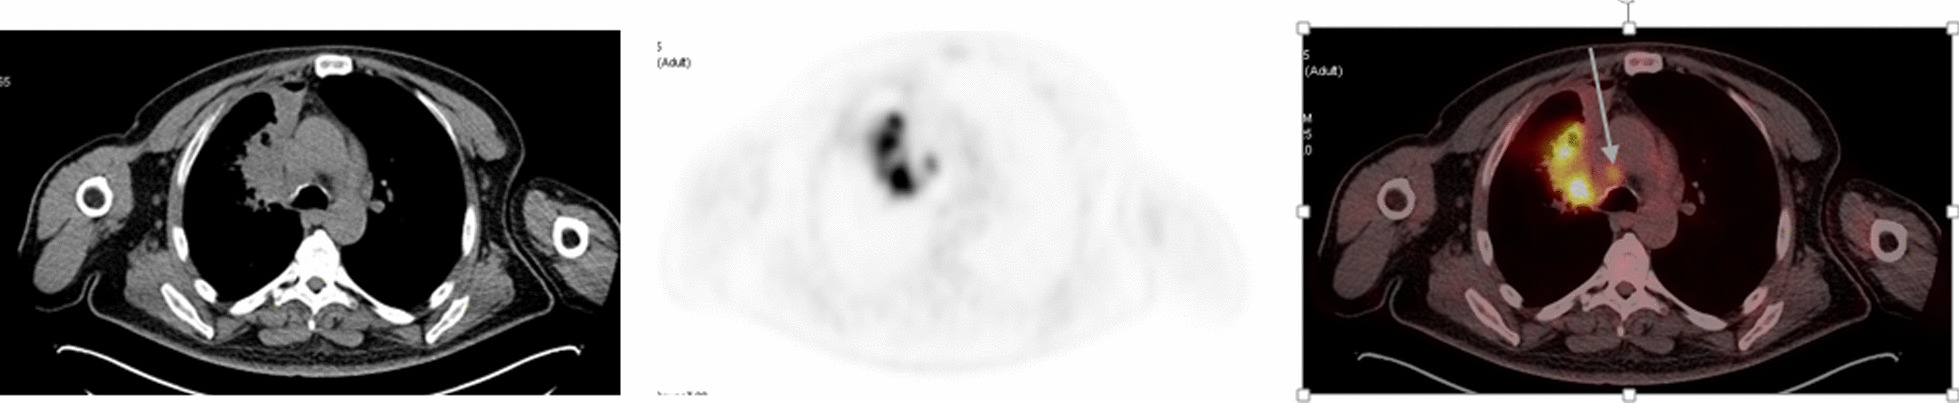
True positive FDG-PET/CT and true positive EBUS/TBNA. A case of moderately differentiated lung adenocarcinoma in the right lung. Axial CT showed prominent pretracheal lymph node. Axial FDG-PET and FDG-PET/CT showed hypermetabolic (SUVmax: 3.8) pretracheal lymph node (arrow) and suggested a metastases. EBUS/TBNA from this lymph node turned positive for metastases that was confirmed later by lymph node dissection
